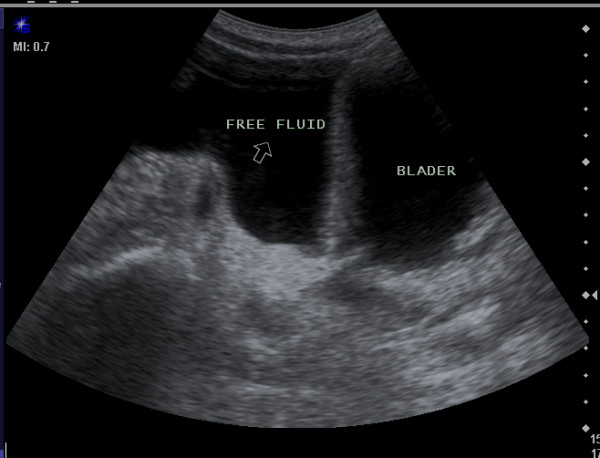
Ultrasonogram revealed free fluid in the paracolic gutter (right) and perisplenic (left).
